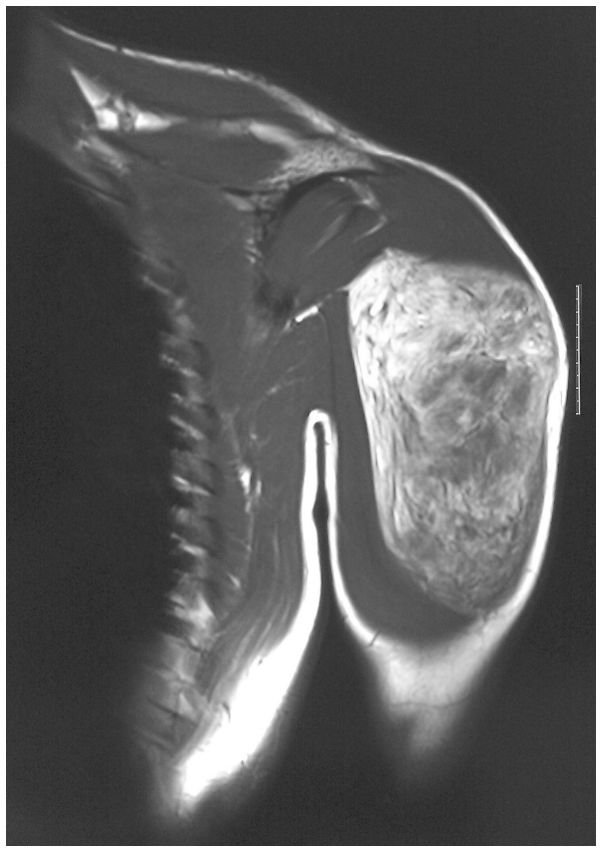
Coronal T1-weighted magnetic resonance imaging of the left shoulder. A large mass is located at the triceps brachii, causing extrusion of the deltoid muscles and teres minor.
